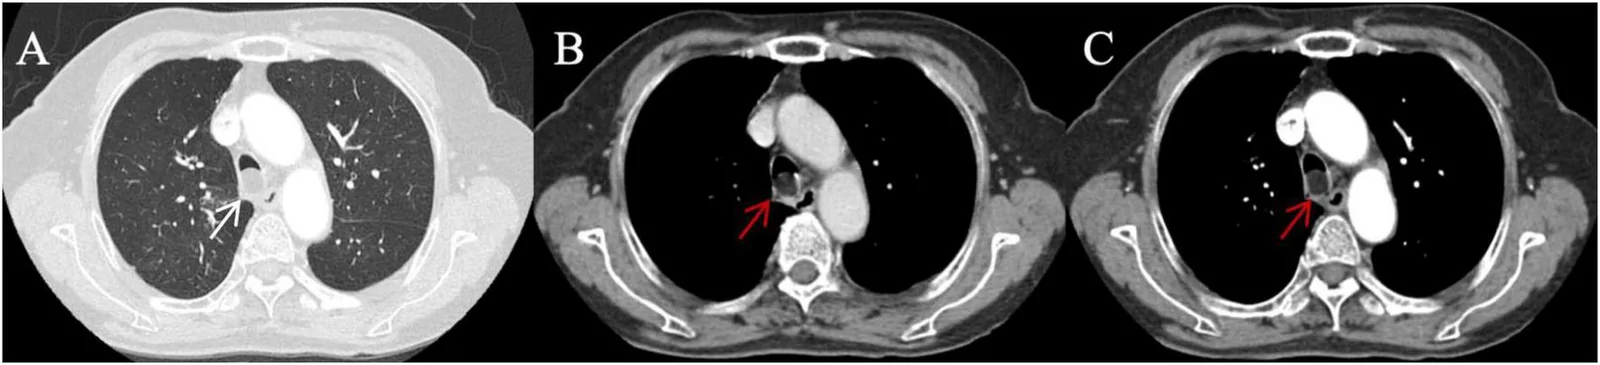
Enhanced chest CT of the tracheal tumor in the lower third: (A) non-contrast CT (B) venous-phase contrast CT (C) arterial-phase contrast CT.
